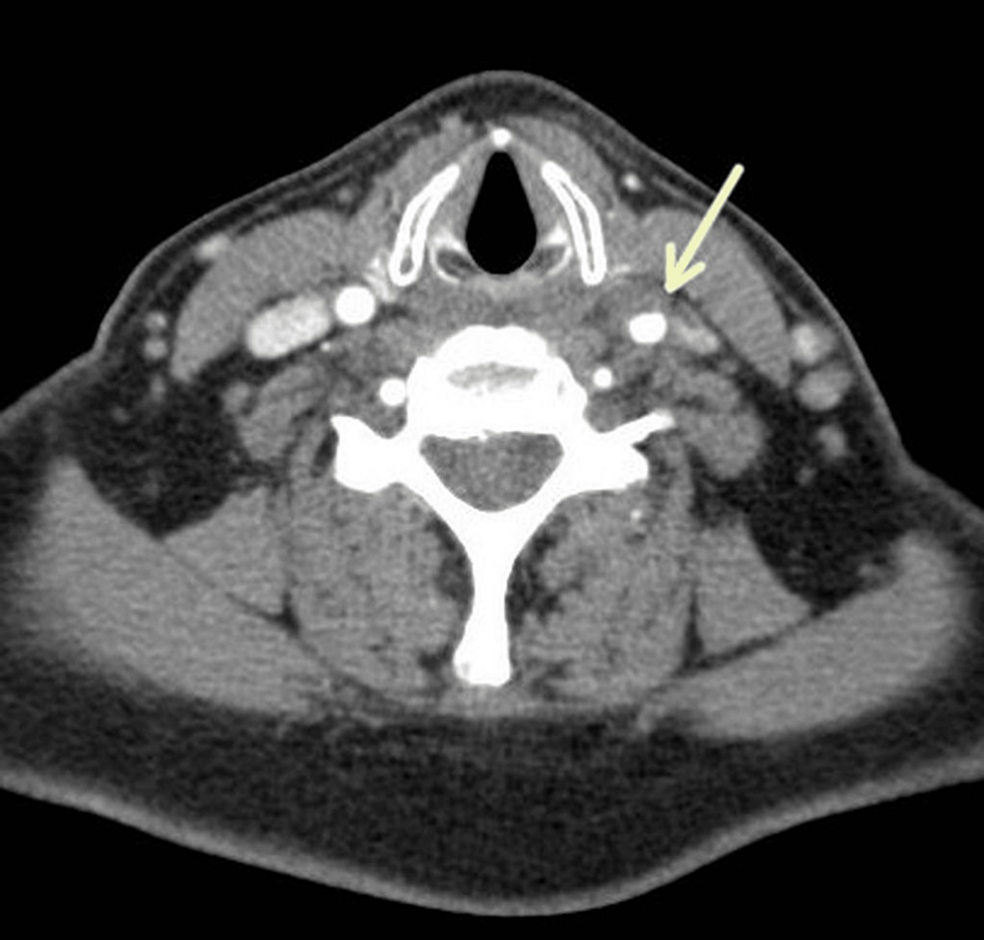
Axial contrast-enhanced CT image showing left common carotid artery reduced caliber (arrow).
